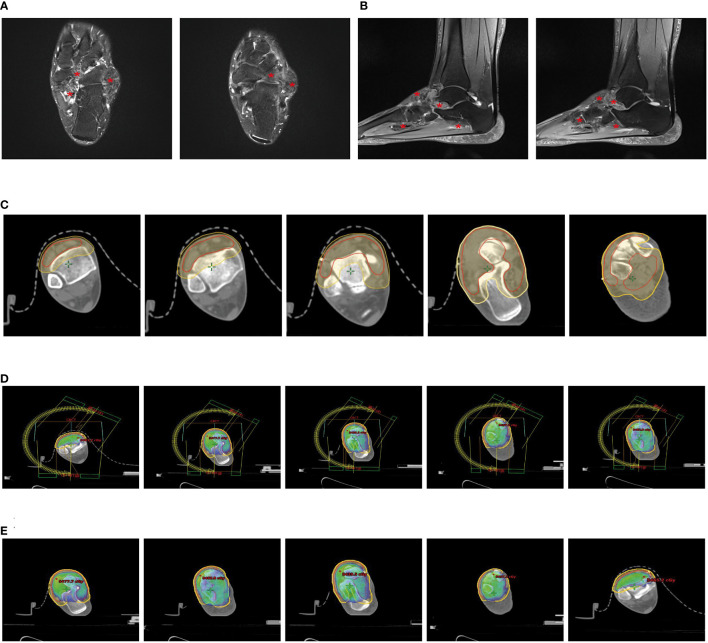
Partial clinical data of a 48-year-old female patient with Tenosynovial Giant Cell Tumor(TGCT). (A, B) MRI shows significant thickening of the synovium in the lateral anterior part of the left ankle and the sole of the foot, with the thickened synovium showing villous and nodular changes. (A) Transverse section. (B) Sagittal section. (C) Target area delineation of the patient’s left ankle joint. (D) Setting of the irradiation field for the patient’s left ankle joint, which is another critical step in the radiation therapy plan, determining the precision and effectiveness of the radiotherapy. (E) Dose distribution in the irradiation field of the patient’s left ankle joint, which helps doctors understand and evaluate the size and distribution of the radiotherapy dose, thus ensuring that patients receive the most effective treatment. # Translation:.
